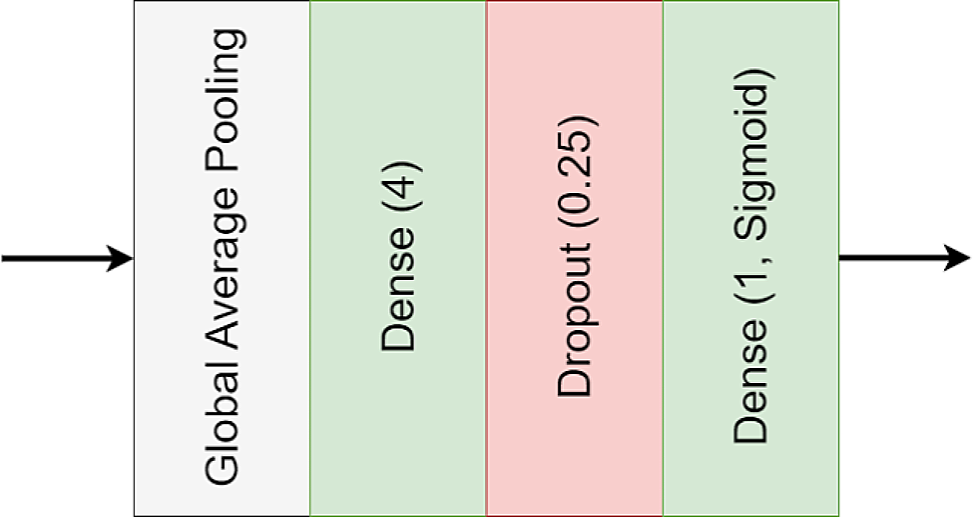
Integration of a new head across four diverse pretrained models, replacing their original heads, for binary classification tasks classifying CT scans into COVID-19 and normal classifications
